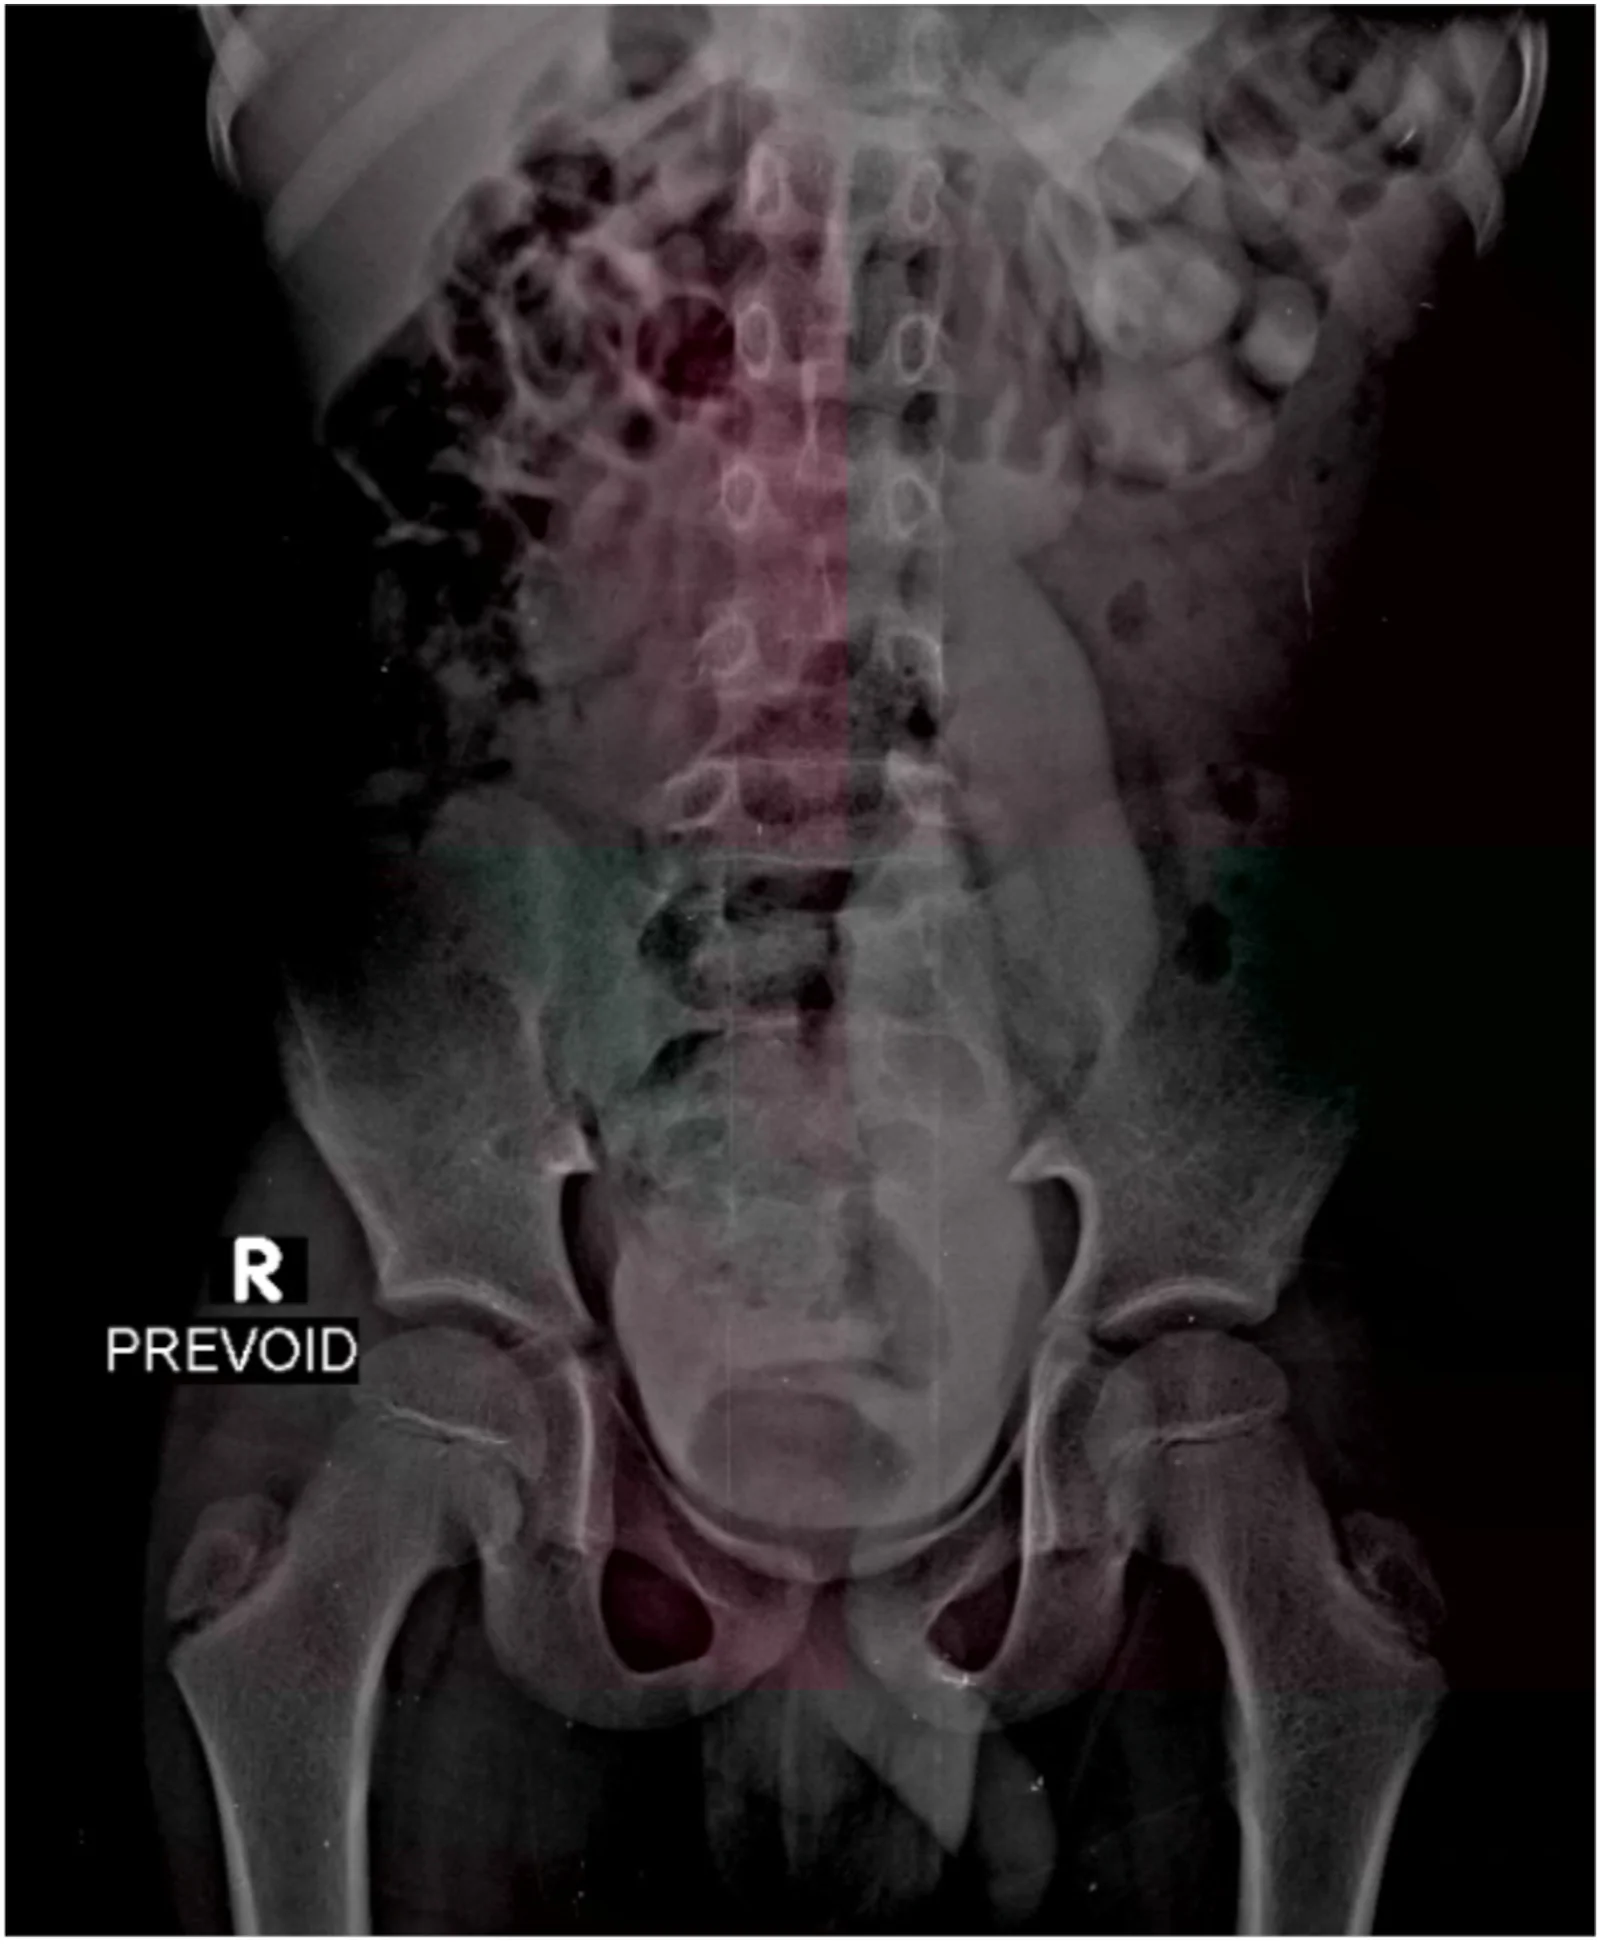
Prevoid film of Xray IVU demonstrating moderate left hydronephrosis with delayed contrast excretion.
